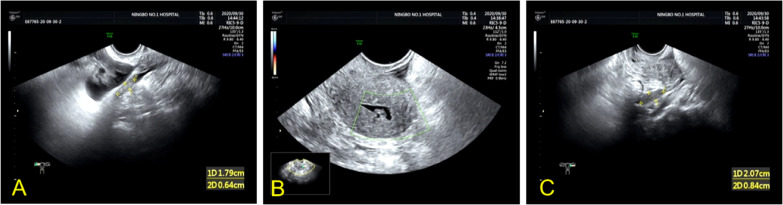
Transvaginal ultrasound images of the uterus and bilateral adnexa of the patient. (A, C) Atrophic right and left ovaries can be visualized. (B) TVU image revealing an atrophic uterus without intimal thickening.
